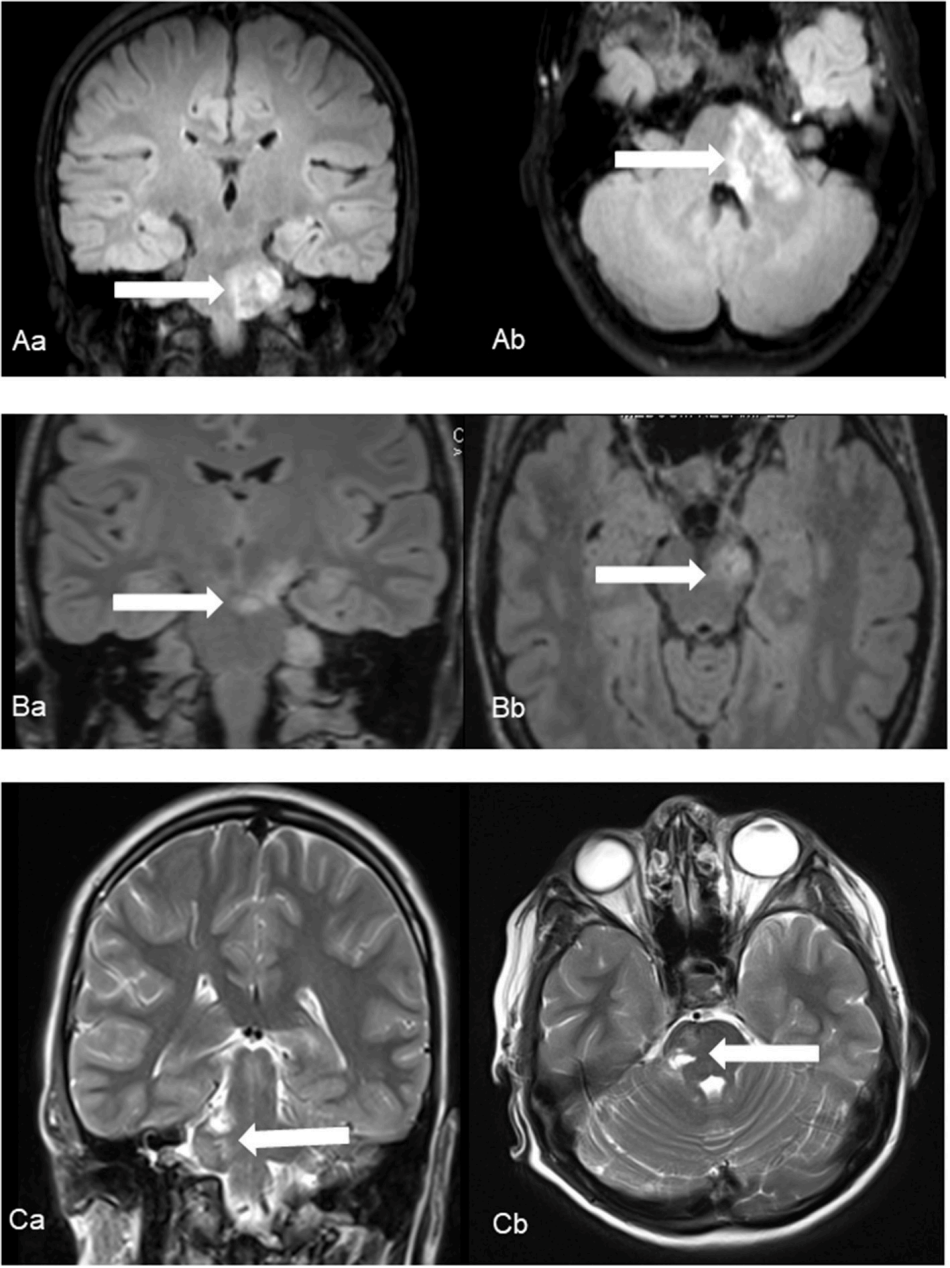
Ischemic events in patients No. 1 (A), No. 2 (B) and No. 3 (C) using coronal (a) and axial (b) fluid-attenuated inversion recover (Patient No. 1 and 2) and T2-weighted (Patient No. 3) images. Characteristic foci of increased T2 intensity (arrows) are identified in the left ponto-medullary transition/cerebellar peduncle (Patient No. 1), in the left cerebral peduncle (Patient No. 2) and in the right pons (Patient No. 3).
